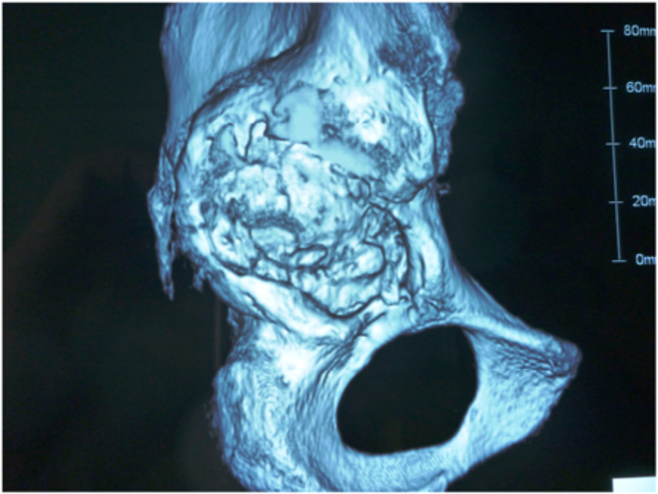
Three-dimensional computed tomographic scan showing American Academy of Orthopedic Surgeons type II cavitary bone defect of the acetabular roof.
